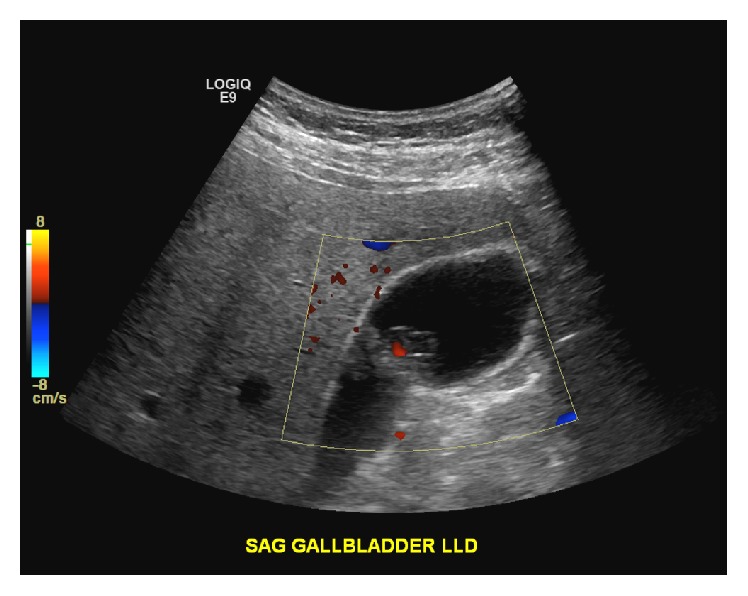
Ultrasound Doppler showing gallbladder mass with high blood flow.
